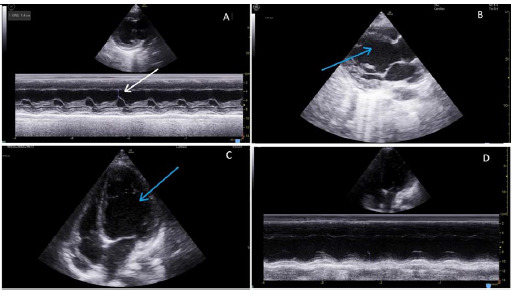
Two-dimensional echocardiographic (2D Echo) findings showing a high E-point septal separation (EPSS) of 1.4 cm (white arrow) (A), a dilated left ventricle (blue arrows) (B, C), and a reduced mitral annular plane systolic excursion (MAPSE) of 8 mm, all of which are suggestive of severe left ventricular systolic dysfunction (D).
